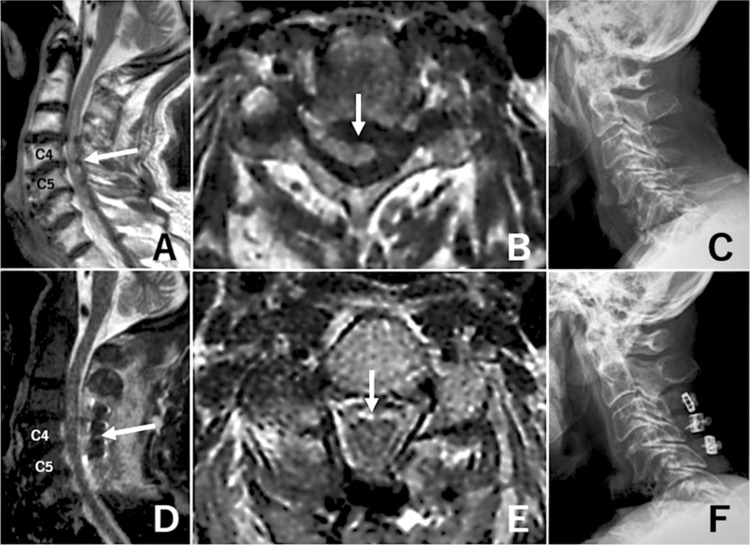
Magnetic resonance images (MRI) and X-ray photographs (A-C) before and (D-F) after surgery. T2-weighted MRIs showing severe cervical cord compression at the C3/4, C4/5, C5/6, and C6/7 levels with intracordal hyperintensity changes. White arrows in (A) sagittal view and (B) axial view showing severe cord compression at the C4/5 level. (C) Lateral radiograph showing cervical spondylosis. T2-weighted short-tau inversion recovery MRIs showing released cervical cord compression. White arrows in (D) sagittal view and (E) axial view showing released cervical cord compression at the C4/5 level. Lateral radiograph showing implantation at the levels of C3, C4, and C5 and removal of the C6 lamina (F).
